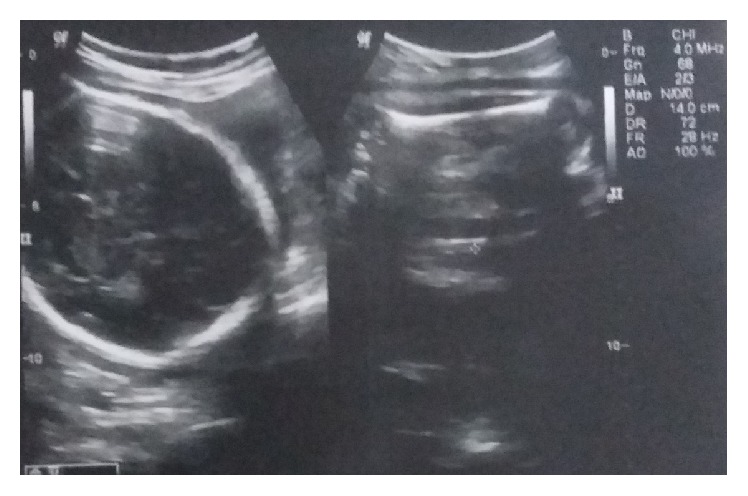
Obstetric ultrasound of the patient.
